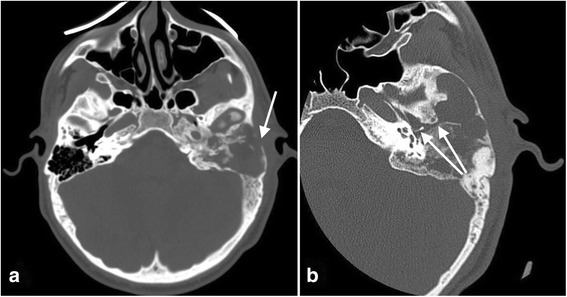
a Axial CT scan of the temporal bone shows massive destruction of the mastoid area with external osteolysis and a fistula in the temporal area. b Axial CT scan at the level of the oval window shows massive fibrous dysplasia involving the temporal bone as well as opacification of the mastoid and middle ear cavity with ossicles destruction and stenosis of the external auditory canal
